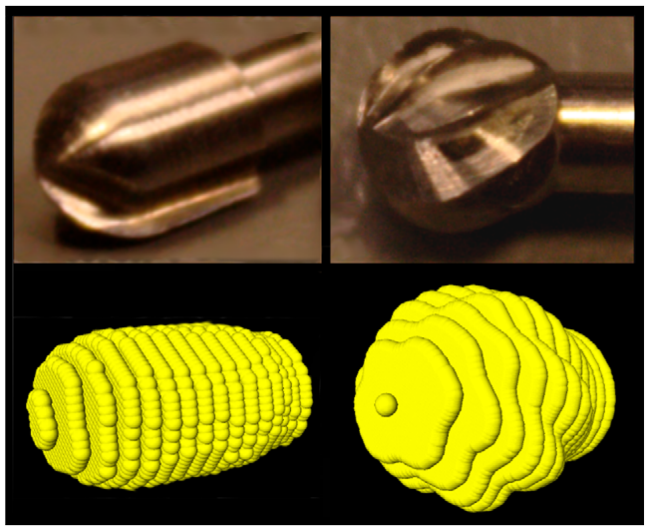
Virtual Drill Bits. Drill bits were scanned with high resolution CT and represented as 3D point clouds. On the left is displayed a 3 mm match-head drill bit and on the right a 4 mm drill bit can be seen.
